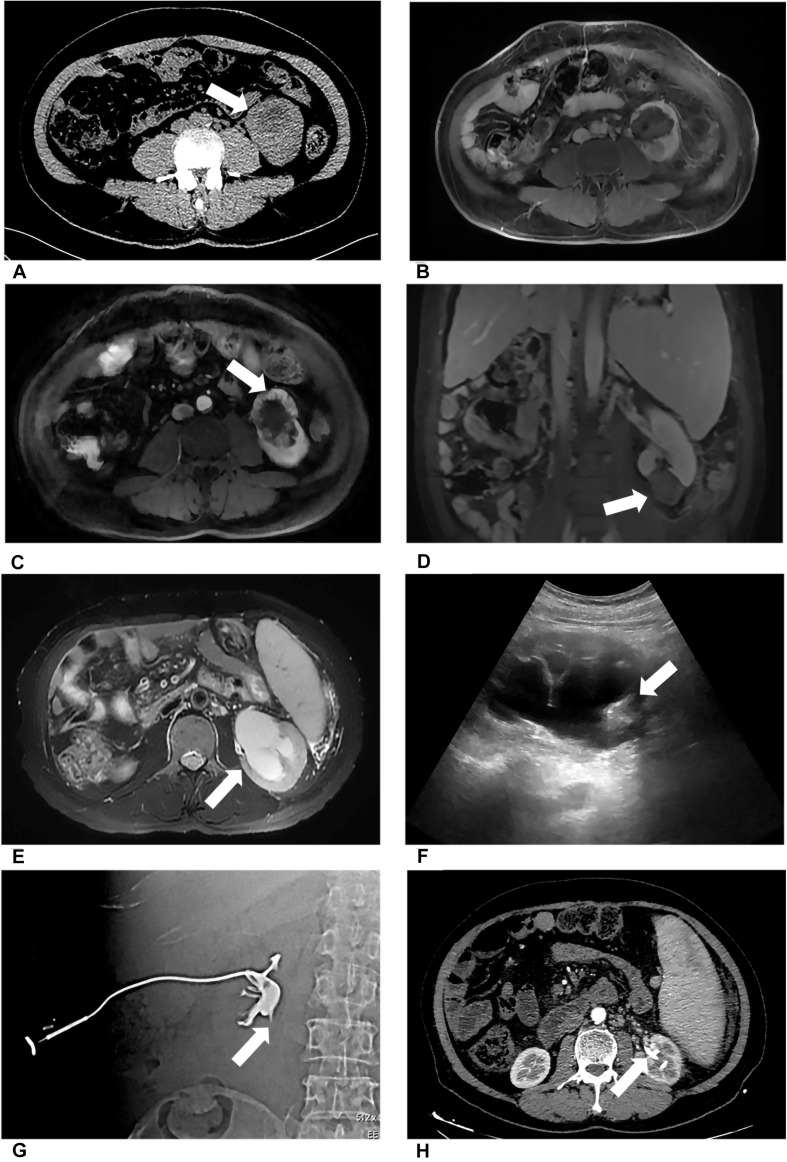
Patient 1 underwent MWA of a 3.6-cm renal tumor in the lower left kidney. 8 months after first MWA, local recurrence was confirmed by MRI, and the patient accepted second MWA. 13 months after second MWA, severe hydronephrosis was detected on his left kidney; the drainage tube was placed in his renal pelvis under US guidance. Digital subtraction angiography (DSA) confirmed serve ureteropelvic junction stricture. (A) Axial enhanced CT shows a renal mass (white arrow) in the lower pole of the left kidney. (B) Axial MRI shows the ablation site (white arrow) 13 days after MWA. (C) Axial MRI shows local recurrence (white arrow) 8 months after the first MWA. (D) Coronal MRI scan obtained in the third day after the second ablation (white arrow). (E) Axial MRI shows severe hydronephrosis (white arrow) 13 months after the second ablation. (F) US image shows the drainage tube (white arrow) after percutaneous puncture catheter drainage under US guidance. (G) DSA shows the contrast agent cannot flow through the ureter in the prone position, considering ureteropelvic junction stricture (white arrow). (H) Axial CT shows the drainage tube (white arrow) in the renal pelvis and without hydronephrosis 3 months after percutaneous puncture catheter drainage.
